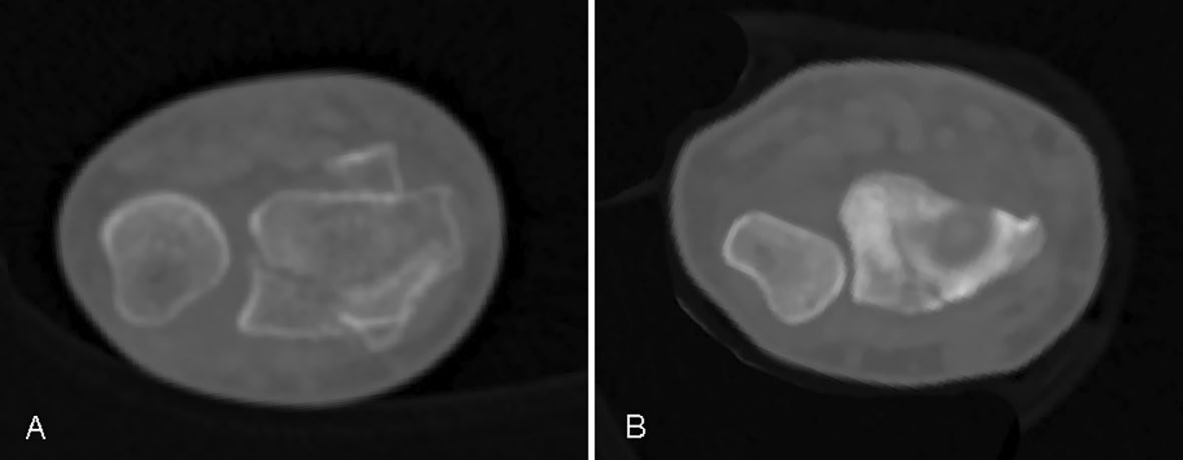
If fracture lines or steps were found on CT images at the site of the sigmoid notch, it was considered as positive (A) for involvement; otherwise, it was negative (B).
